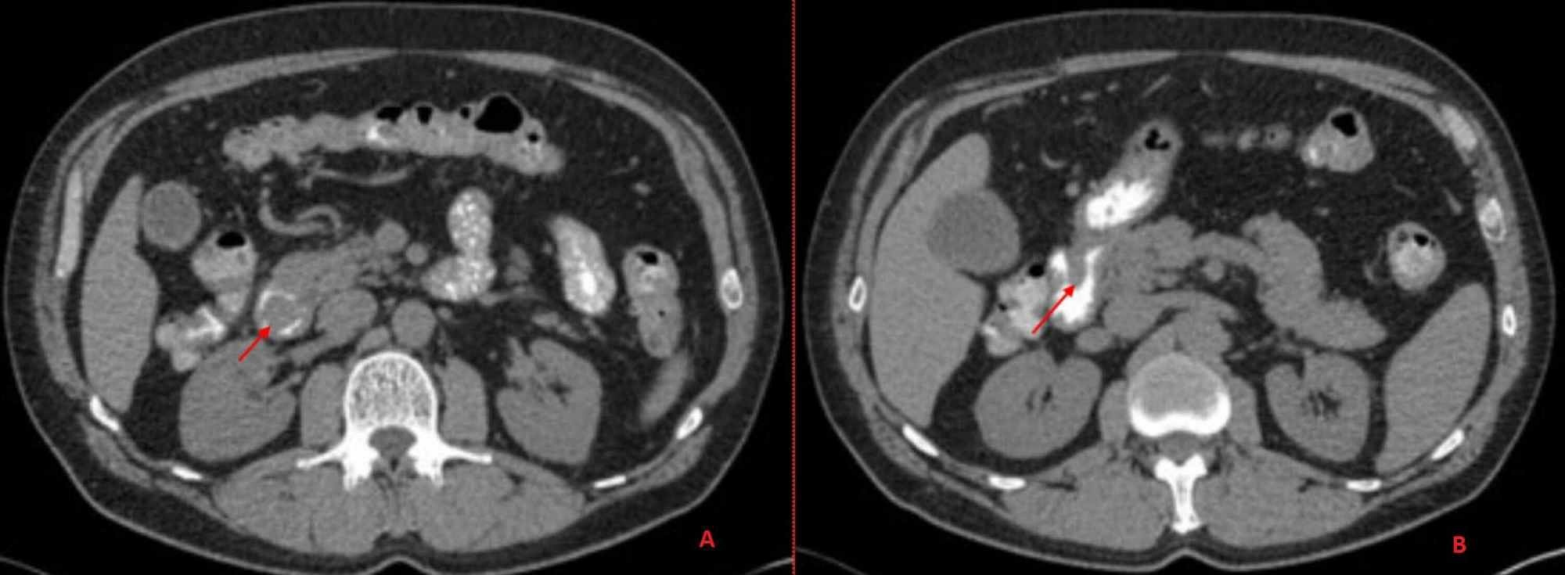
A CT abdomen with contrast shows an elongated, well-circumscribed, intraluminal mass, arising from the duodenal bulb, measuring 5.3 x 1.7 x 1.5 cm.
